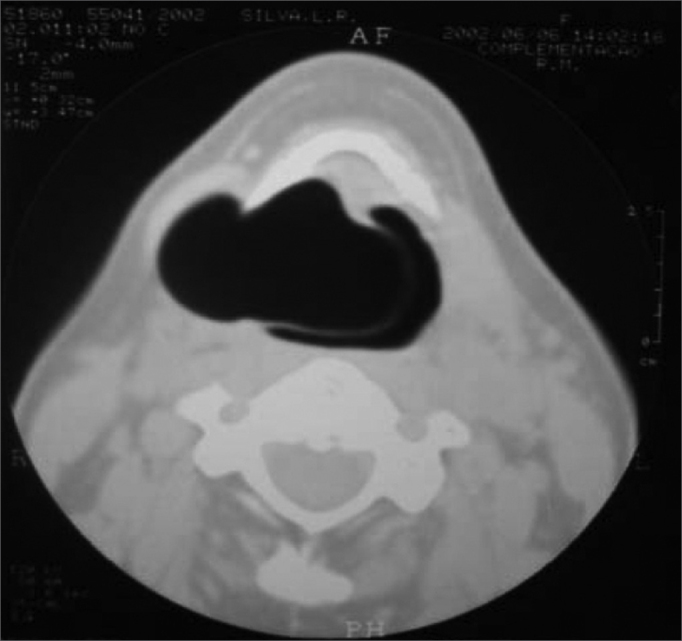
Larynx CT scan showing the laryngocele.
